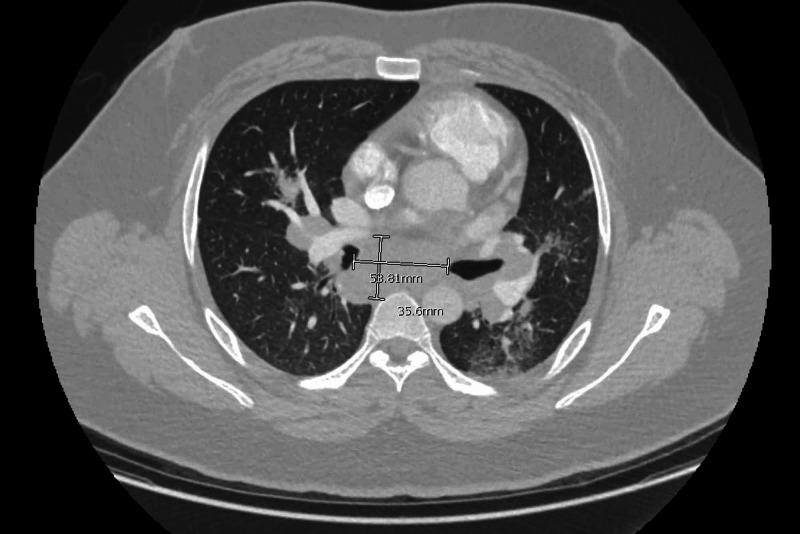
CT angiography chest transverse view showing enlarged subcarinal lymph node measuring 3.7 cm × 5.4 cm (dimensions marked in white).
